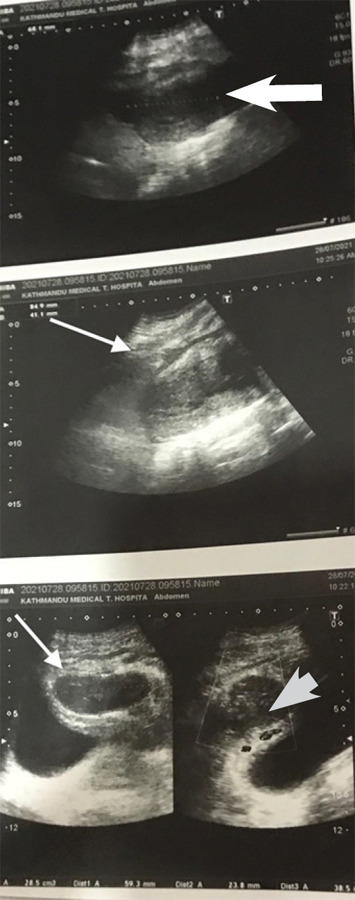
Ultrasonographic images showing uterus and uterovesical collection.
